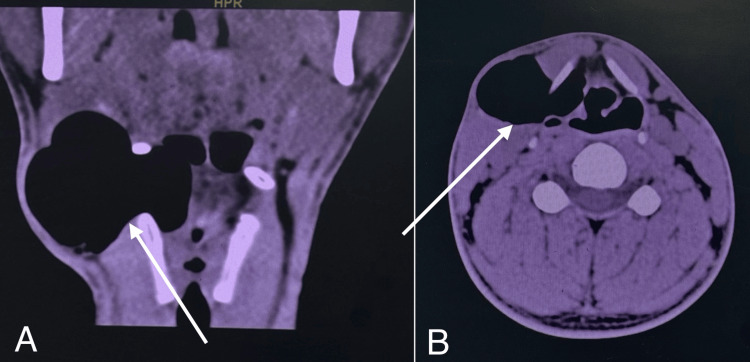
Non-contrast CT of the neck showing (A) coronal view with a right-sided air-filled swelling arising from the laryngeal saccule and extending through the thyrohyoid membrane, and (B) axial view confirming its lateral extension.
